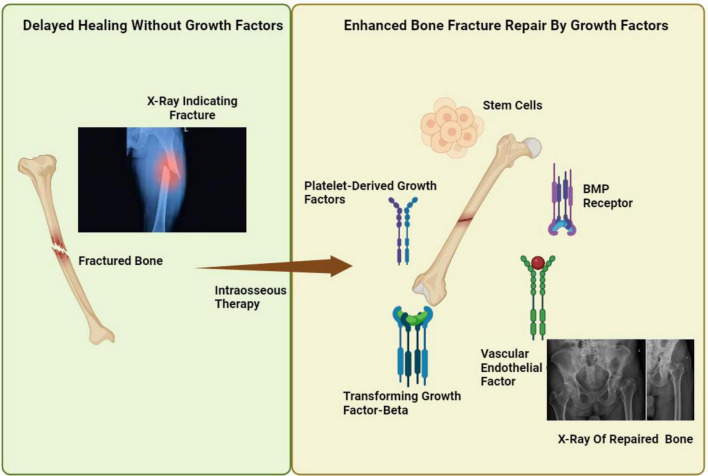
Growth factor-mediated enhancement of bone fracture healing through stem cell activation and signaling pathways, figure contrasts delayed bone healing without growth factors with enhanced repair through intraosseous therapy, highlighting the efficacy of platelet-derived growth factors, BMP Receptors, and Transforming Growth Factor-Beta in conjunction with stem cells, as evidenced by pre- and post-treatment X-rays.
